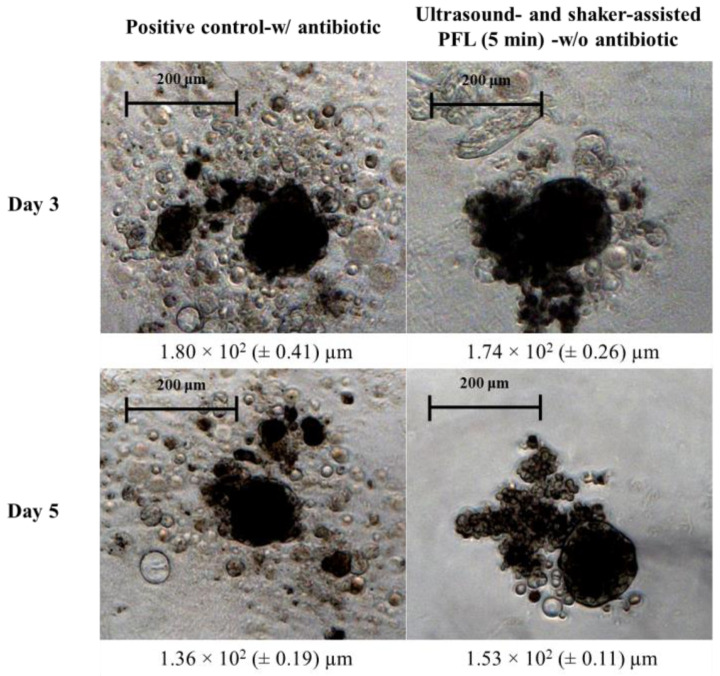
Spheroid culture (3D) derived from mesenchymal stem cells (MSCs) for day 3 (top row) and day 5 (bottom row). In the left column, the cells derived from the positive control (with antibiotics) and the spheroids appear dense and compact, demonstrating strong cell–cell adhesion. The right column shows the formation of spheroids of cells after ultrasound- and shaker-assisted PFL treatment protocol (for a treatment of 5 min). The spheroids demonstrated comparable diameters to the positive control. The Feret diameter is indicated below each image (*n* = 5).
